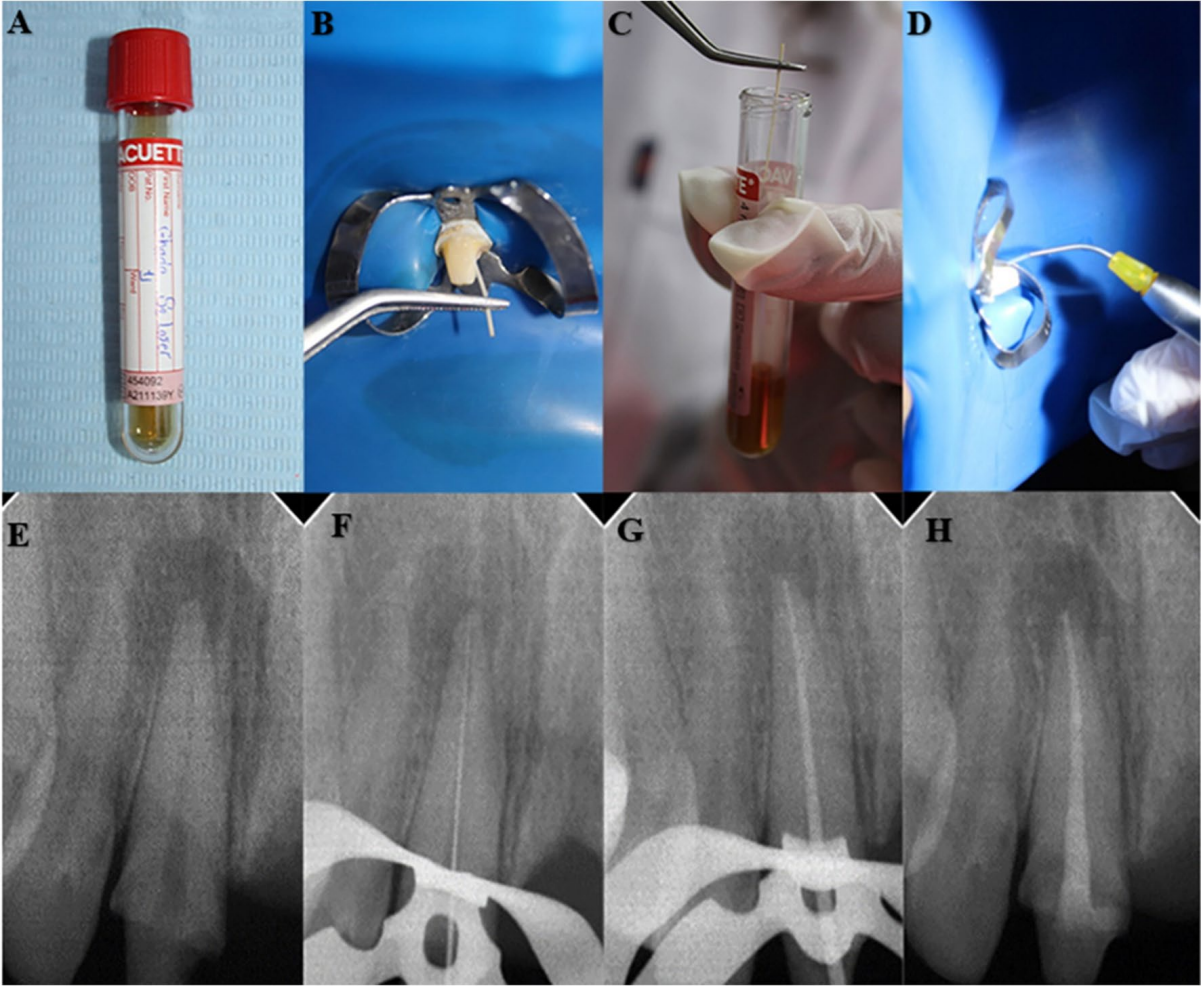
Sterile tube containing Thioglycolate, (B) Paper point is inserted inside the root canal, (C) The paper point is then inserted inside the sterile tube, (D) laser is applied inside root canal, (E) Preoperative periapical radiograph is taken, (F) Working length is confirmed with k-file #15, (G) Periapical radiograph is taken with the master apical cone in place, (H) Postoperative periapical radiograph is acquired
